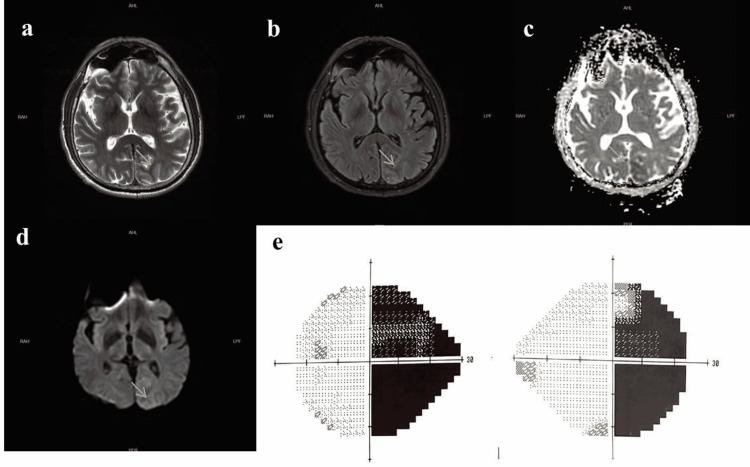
Patient's MRI and visual field test MRI showed a non-space-occupying T2 (a) and fluid-attenuated inversion recovery image (FLAIR) (b) sequences hypointense lesion of the subcortical white matter in the left occipital lobe (arrows). Apparent diffusion coefficient (ADC) signal (c) significantly decreased (arrow), diffusion-weighted imaging (DWI) signal (d) showed subtle gray matter hyperintense and white matter hypointense lesion (arrow). The visual field test was consistent with the patient’s right homonymous hemianopia (HH) (e).
